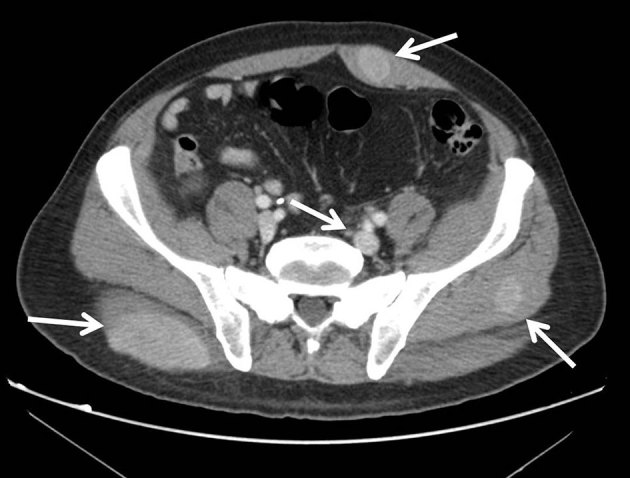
Upper abdominal CT scan after i.v. contrast showing multiple retroperitoneal masses afecting both adrenal glands, left kidney, retrocrural space, intraperitoneal and retroperitoneal fat (arrows).
